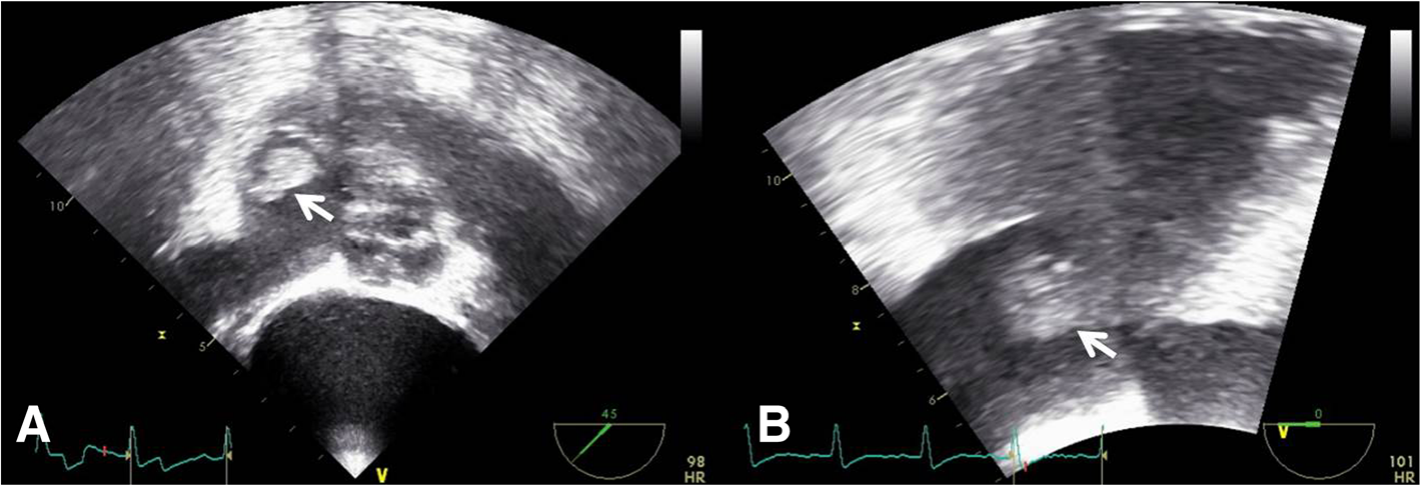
a and b Transesophageal echocardiography revealing a globular, mobile, echogenic mass (1.91 × 1.0 cm; *arrows*) attached to the tricuspid valve
